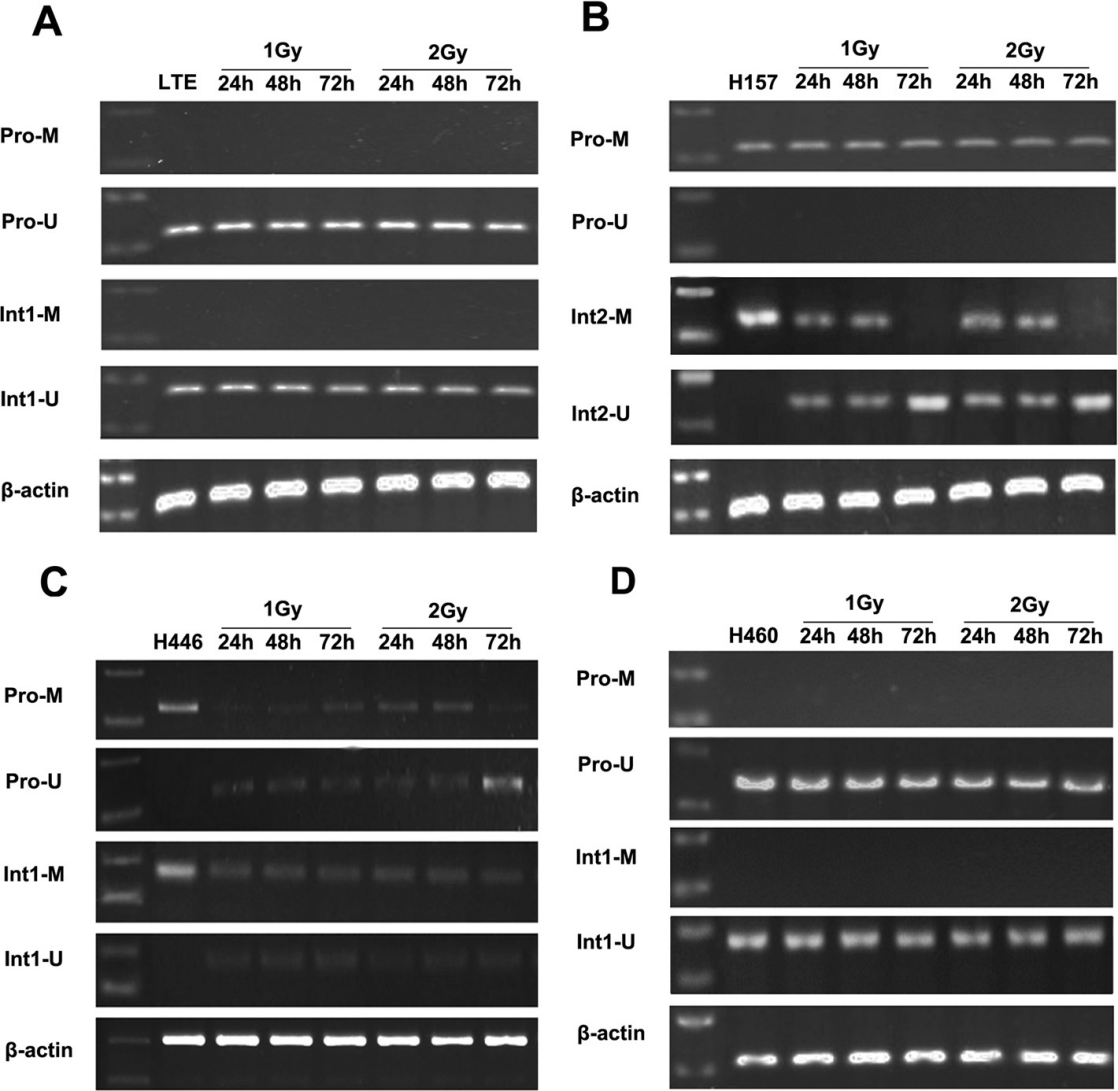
The effect of X-ray irradiation on methylation status of the Axin gene in lung cancer cell lines. There is no obvious change in unmethylated status of the Axin promoter and first intron in the LTE (A) and H460 (D) cell lines after X-ray irradiation, while significant demethylation could be detected in the second intron of H157 cells (B) and the promoter and first intron in H446 cells (C).
